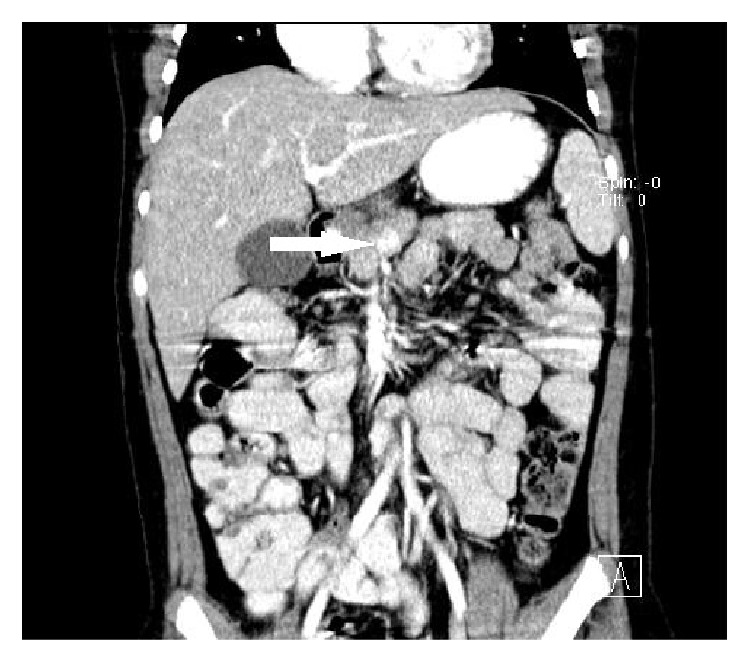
Abdominal CT scan (coronal view) shows intrapancreatic tumor (arrow).
